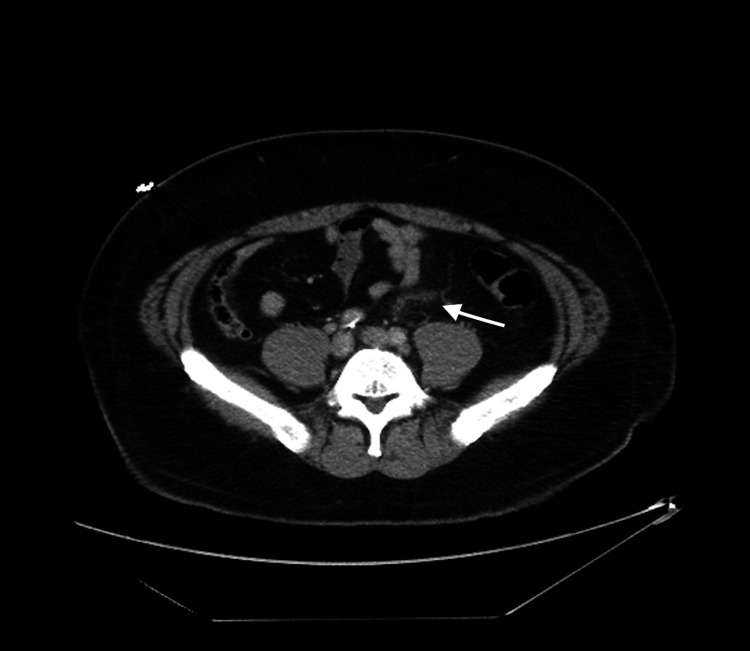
Transverse view CT abdomen and pelvis with delayed imaging to capture venous phase showing (arrow) inflammation surrounding the inferior mesenteric vein concerning for thrombophlebitis. CT, computed tomography.
